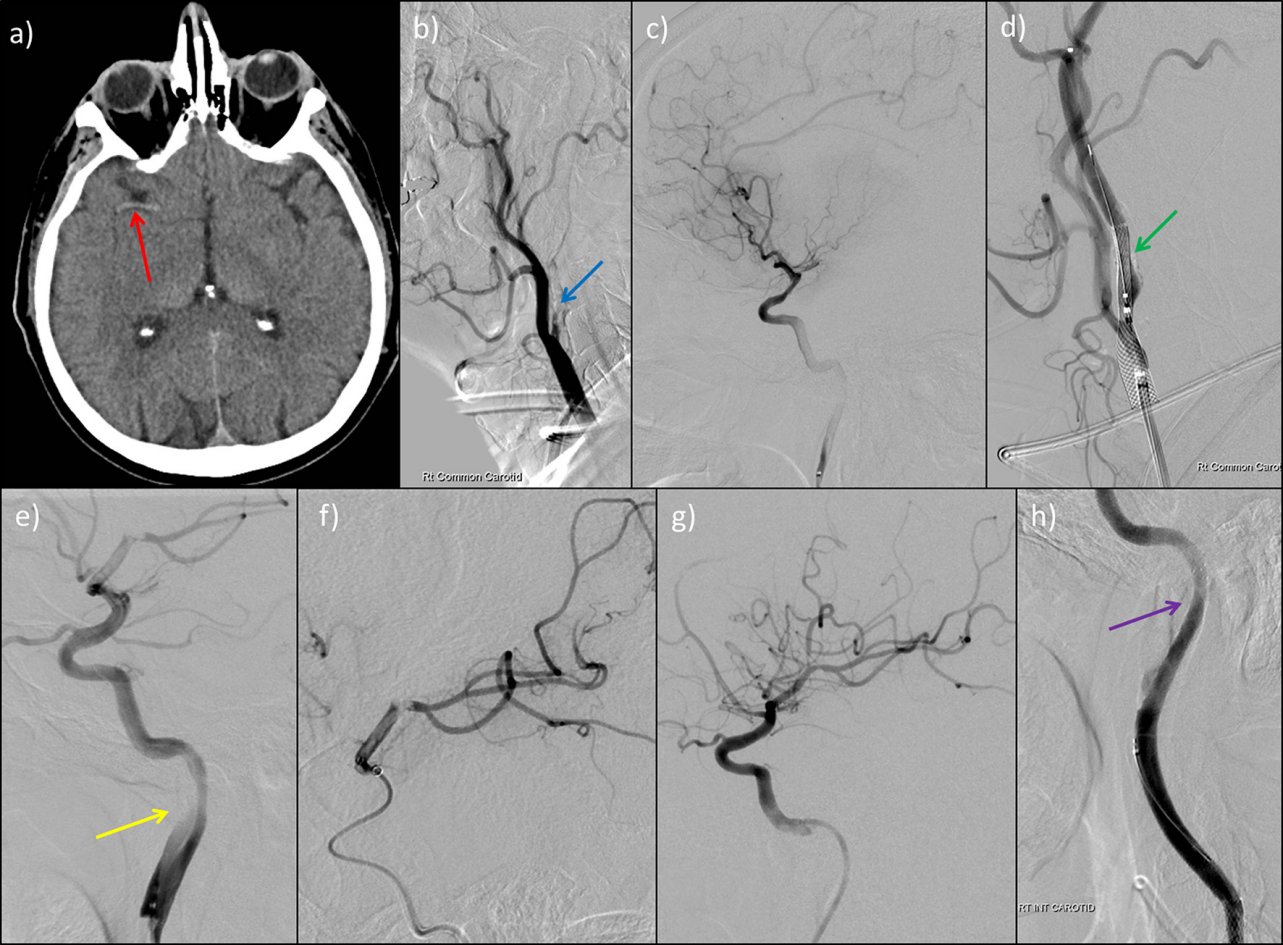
Plain CT scan of head (a) and prethrombectomy (b, c), during thrombectomy (d, e, f) and post-thrombectomy (g, h) digital subtraction angiogram images in a 61-year-old man who presented with a 10 min seizure, followed by left-sided weakness and neglect. Plain CT scan of head shows hyperdense thrombus in the right middle cerebral artery (red arrow, a) with angiogram identifying a critical stenosis of the internal carotid artery origin (blue arrow, b). We performed middle cerebral artery thrombectomy using stent retriever technique (e and f). An internal carotid artery stent was inserted (green arrow, d) complicated by an iatrogenic dissection (yellow arrow, e) necessitating stenting (purple arrow, h).
